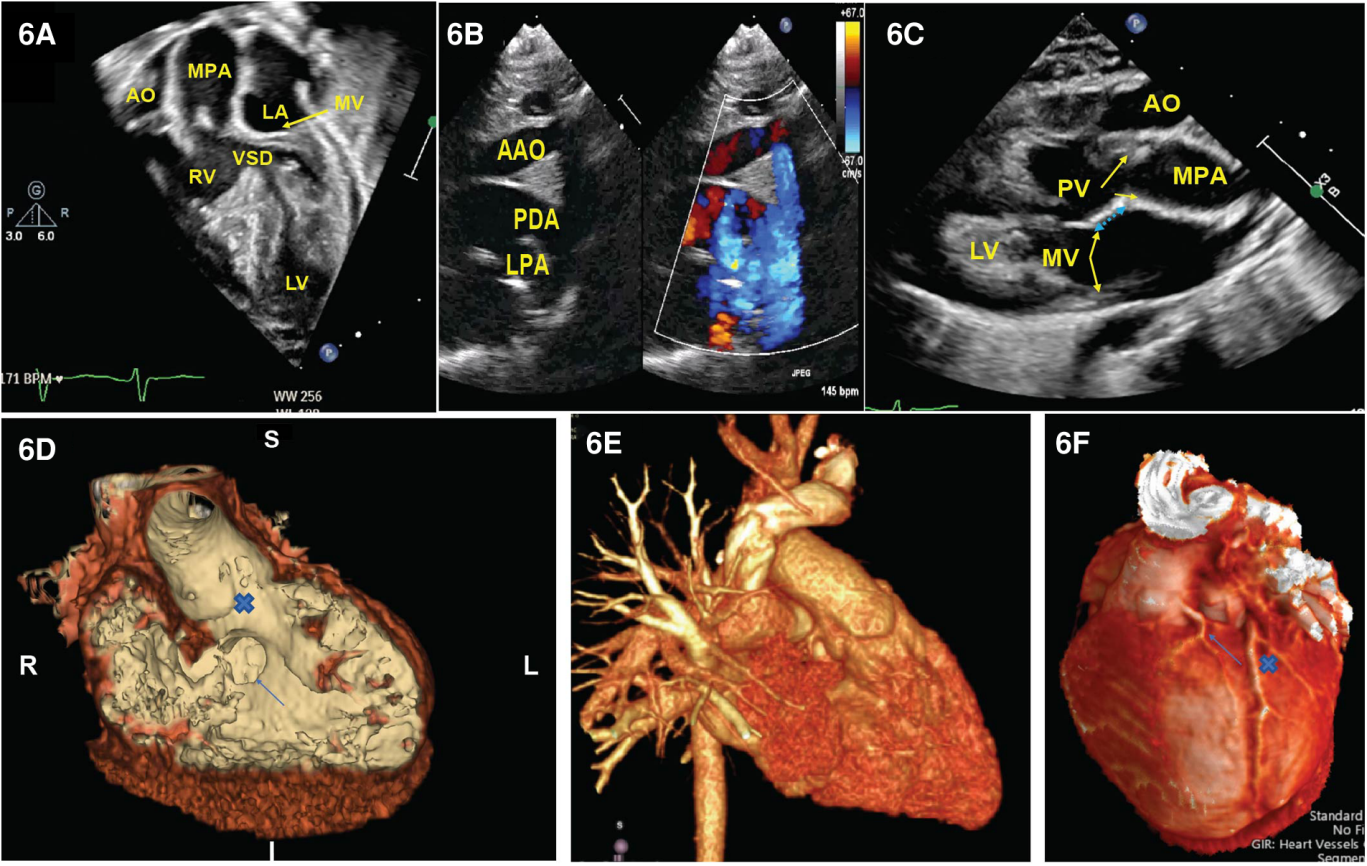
Representative images of DORV. (A) Four-chamber view demonstrated aorta is right to the PA and a VSD is beneath the pulmonary valve. (B) Supra-sternal view demonstrated arch hypoplasia with a large patent ductus arteriosus. In (C), we appreciate a parasternal long-axis view where the aorta is anterior to the PA with loss of the aorto-mitral valve continuity (blue arrow). (D) CT angiogram endoluminal view. A view of the VSD from the right ventricular side (arrow) and the VSD to aortic pathway X. (E) 3D reconstruction of the heart showing the anterior origin of the aorta and the origin of the left coronary artery. (F) Dual LAD arising from the leftward-facing sinus (arrow) that courses down the interventricular groove parallel with the larger retropulmonary LAD (x). LAD: left anterior descending artery.
